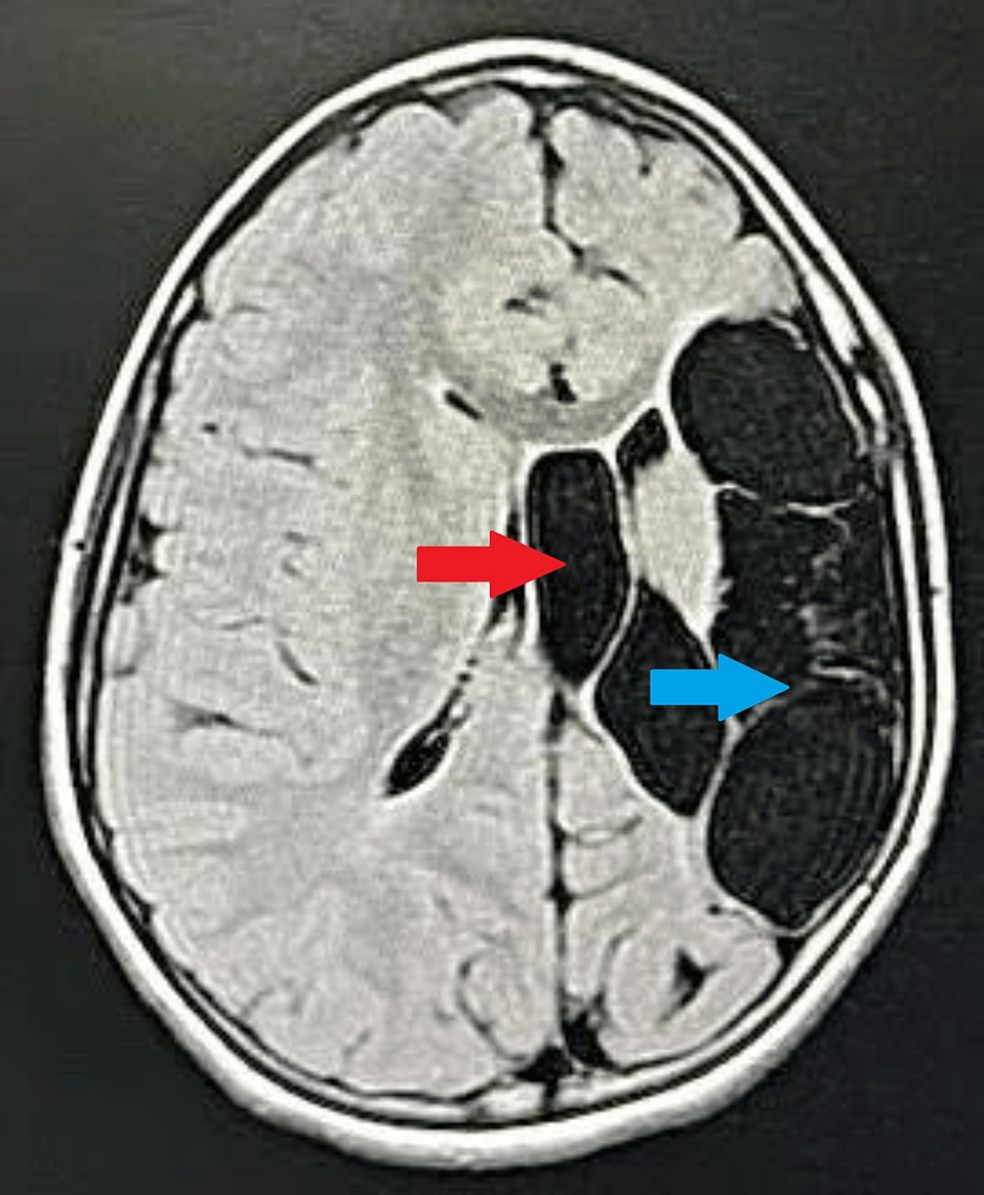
MRI brain axial FLAIR image Altered signal intensity is noted at the frontoparietal lobe on the left side with the loss of gray-white matter junction, encephalomalacia, and gliotic changes with dilatation of anterior horn and body of ipsilateral lateral ventricle appearing hypointense on FLAIR Red arrow: dilated left lateral ventricle. Blue arrow: altered signal intensity at the left frontoparietal lobe FLAIR: fluid-attenuated inversion recovery; MRI: magnetic resonance imaging
